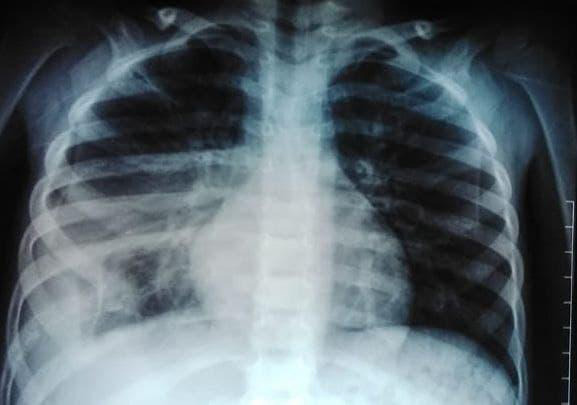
Chest X-ray after two cycles of chemotherapy.
